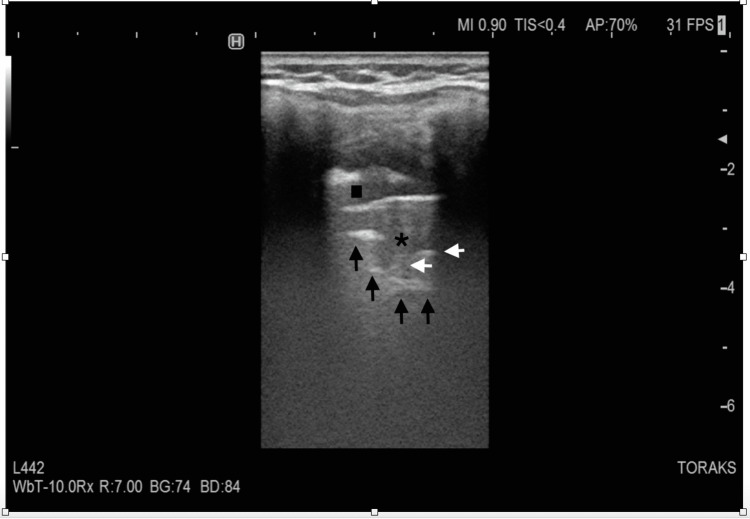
Lung ultrasound of the left upper BLUE point The figure demonstrates no pleural sliding, thickened and severely broken pleural line (square), consolidated areas (asterisks), air bronchograms (white arrows), and the shred sign (black arrows).
